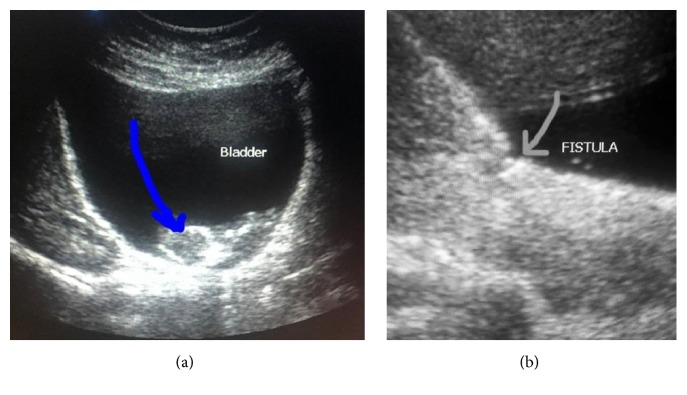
Ultrasound images demonstrate (a) a fetus in the mother's bladder and (b) the fistulous tract between the cavities of the uterus and bladder.
